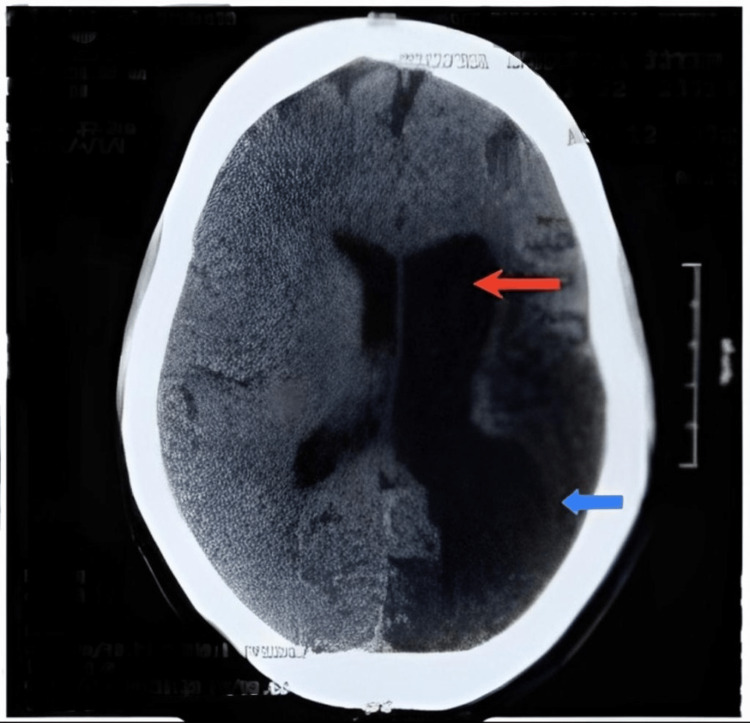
Brain CT scan findings of the patient. The red arrow shows ventricular dilatation of the left lateral ventricle. The blue arrow shows gliotic changes in the temporoparietal and occipital lobes. CT, computed tomography
